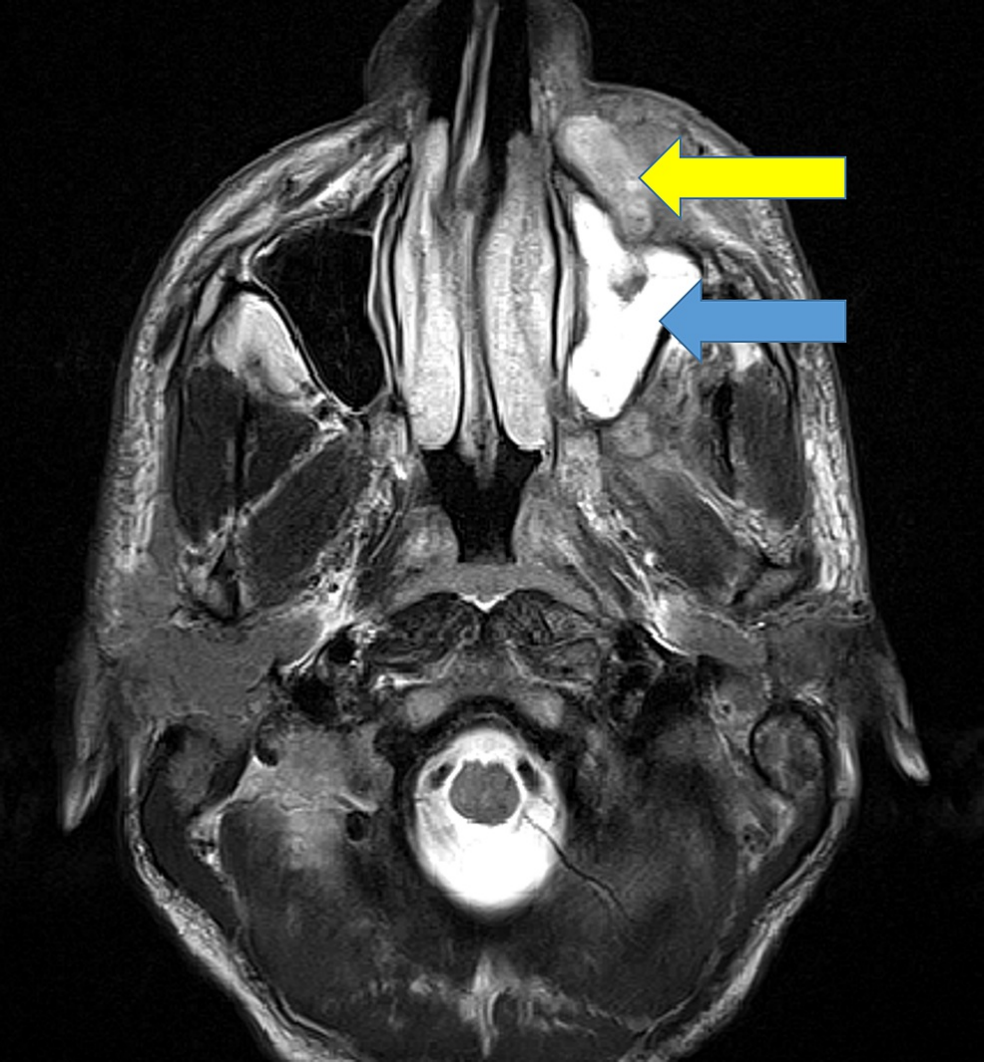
MRI-T2 weighted axial section showing mucosal enhancement of left maxillary sinus (blue arrow) with left cheek abscess (yellow arrow) anterior to the maxillary sinus T: transverse relaxation time
